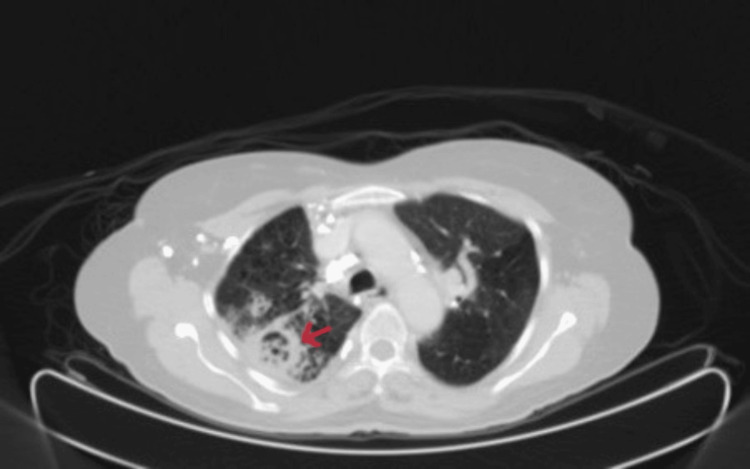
CT component in lung window, showing a cavitary lesion, seen in the posterior segment of the right upper lobe (arrow) CT: computed tomography
